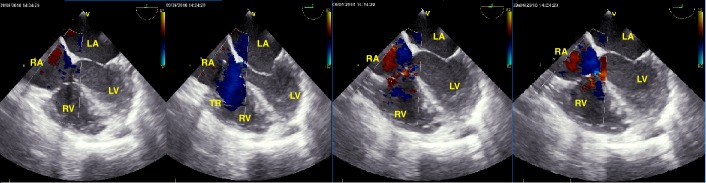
Four-chamber echocardiographic view showing regurgitant jet across the tricuspid valve Blue color signifies regurgitation. TR: tricuspid regurgitation; TV: tricuspid valve; RV: right ventricle; LV: left ventricle; TV: tricuspid valve; RA: right atrium; LA: left atrium
